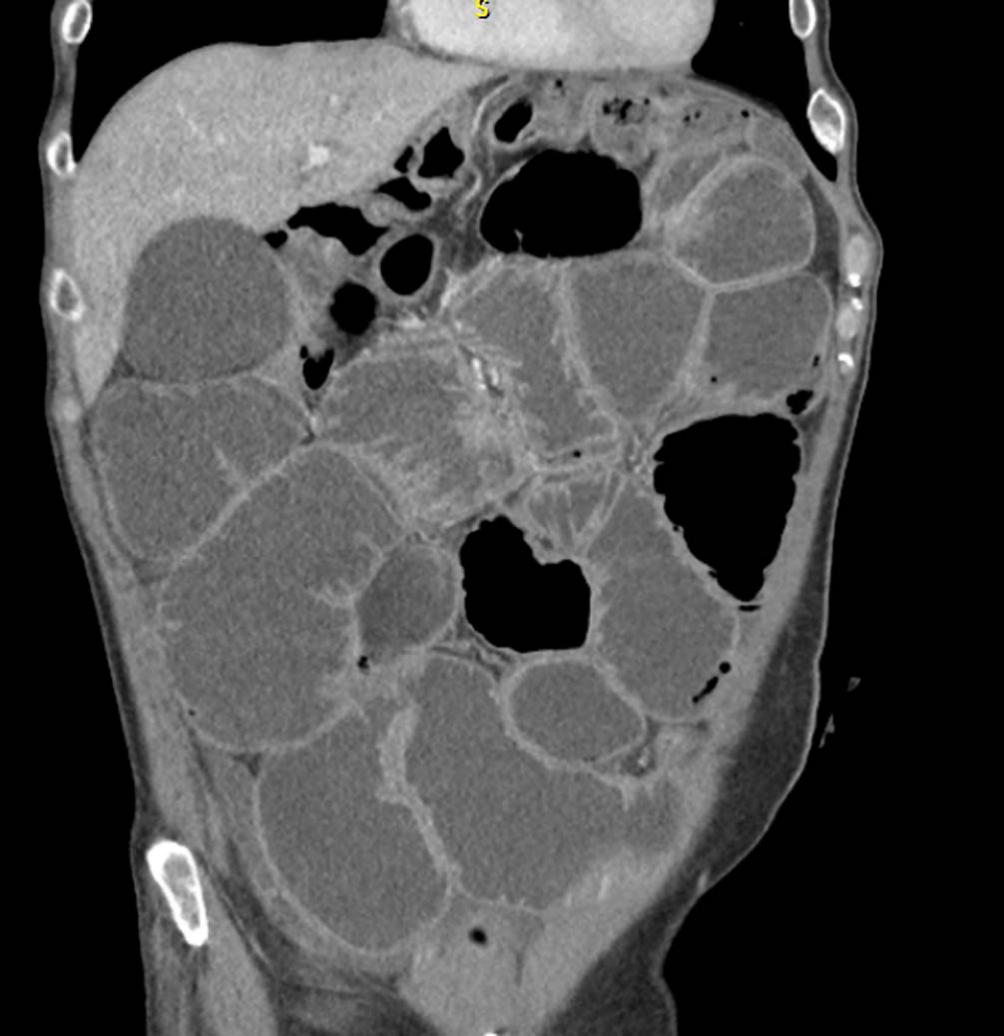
A CT scan showing dilated small bowel without a transition point (suggesting intestinal pseudo-obstruction) in patient with adenocarcinoma of prostate (nonmetastatic).
